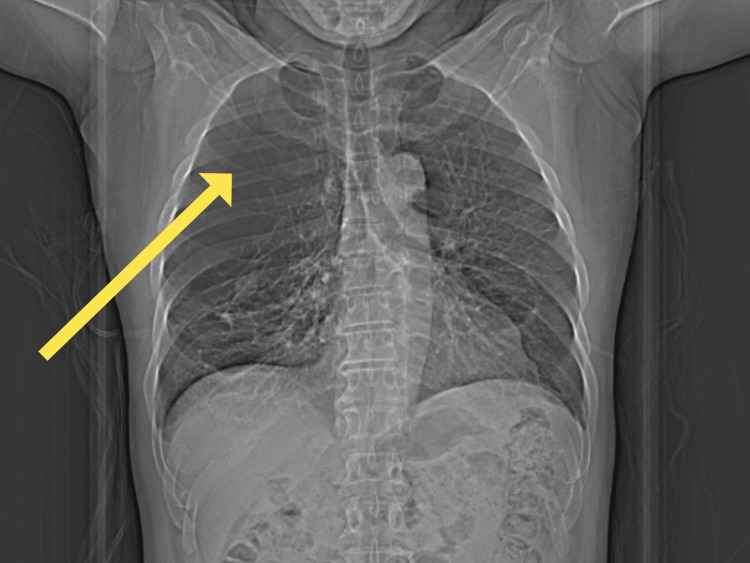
Preoperative chest X-ray demonstrating severe bullous emphysema. The yellow arrow indicates a large lucency in the right upper lung zone consistent with a giant bulla.
